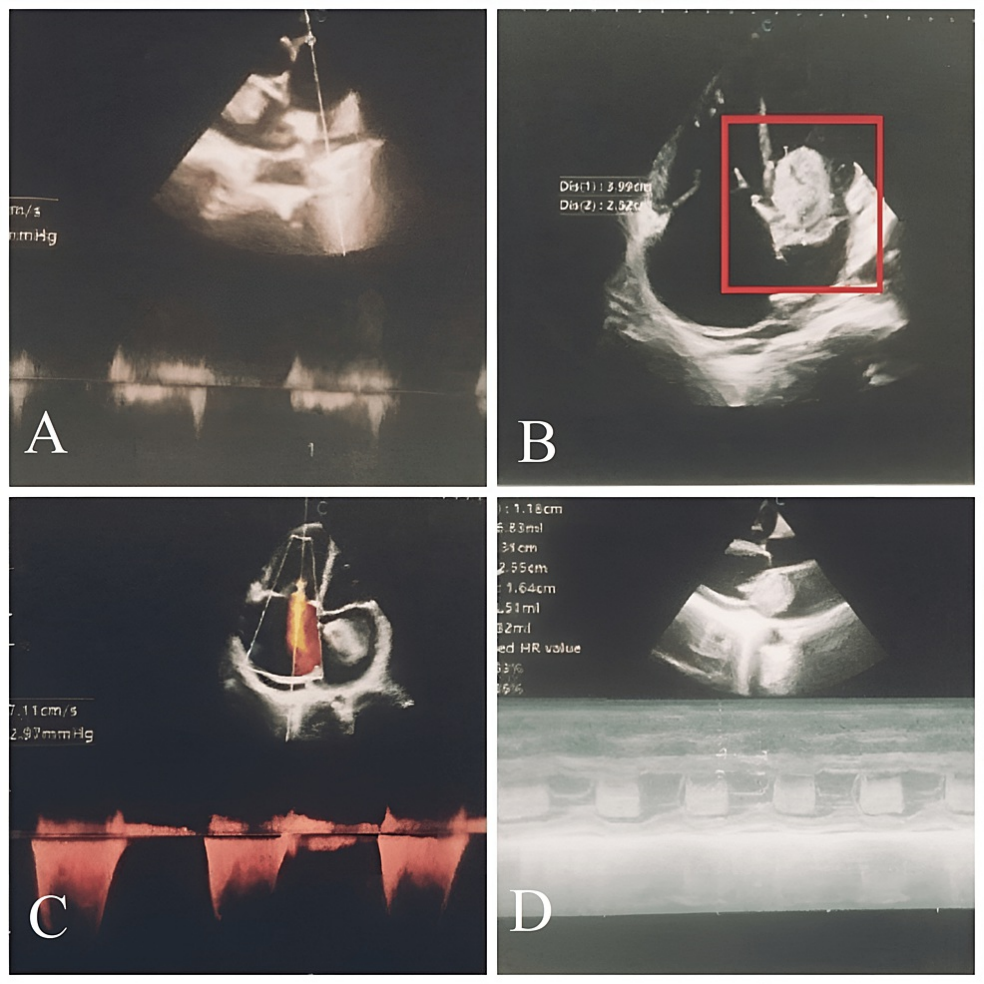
Preoperative echocardiography (A) dilated left atrium; (B) large left atrial mass measuring 3.99 X 2.52 cm, attached in the mid part of the interatrial septum; (C) mild left ventricle inflow obstruction; (D) left atrial mass encroaching left ventricle during systole and causing prolapse of the anterior mitral leaflet
